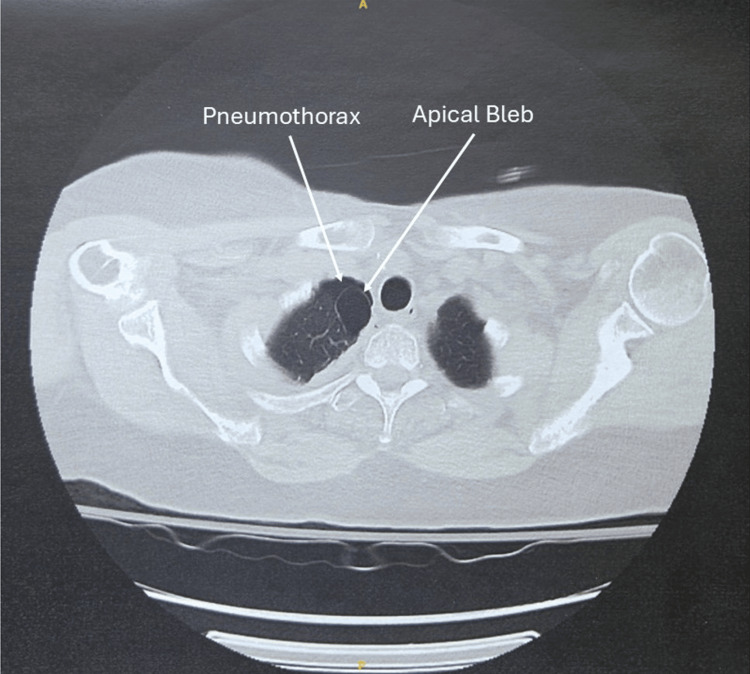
CT chest, axial view, demonstrating a residual small right apical pneumothorax and adjacent 1.7 cm apical bleb
